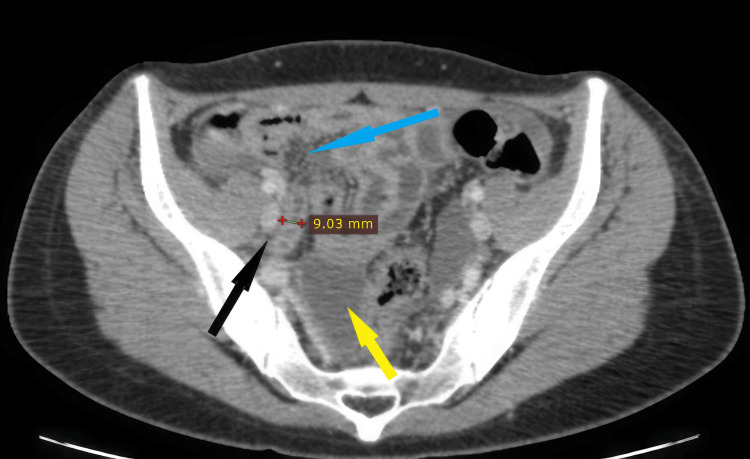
Enhanced CT scan (axial view) showing a thickened appendix (black arrow) with an infiltrated mesenteric fat (blue arrow) and free fluid (yellow arrow).
